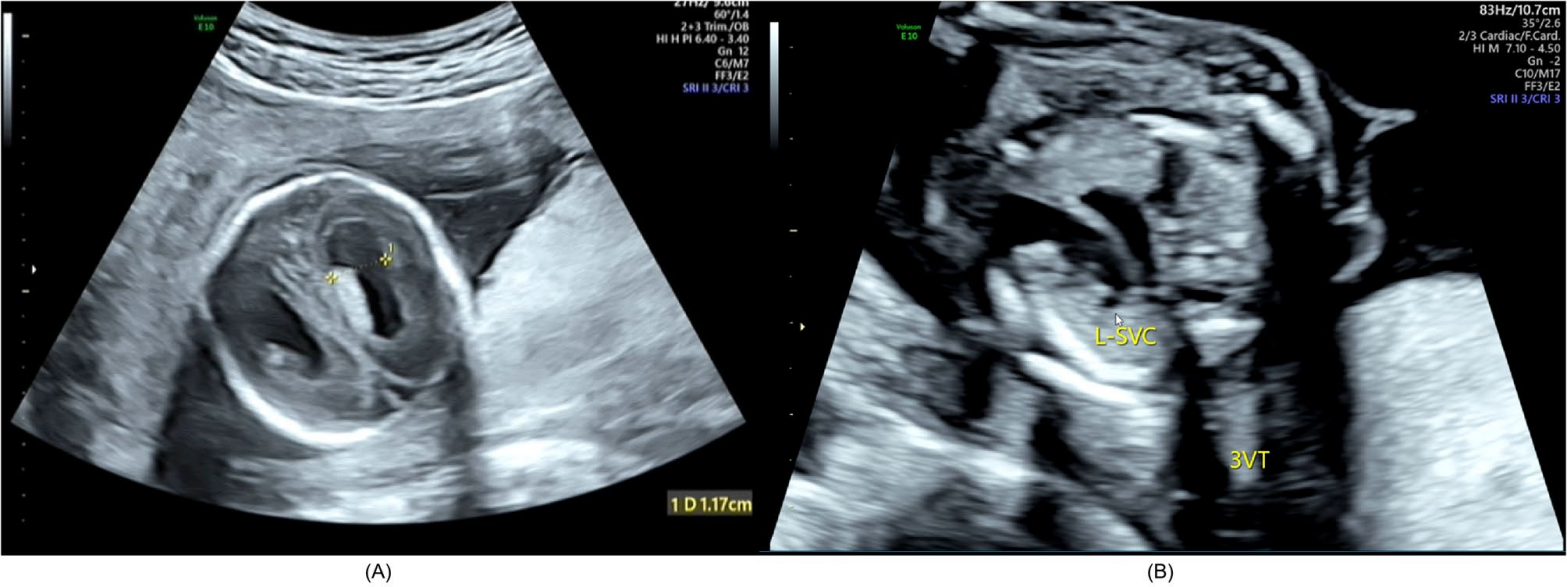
Color doppler ultrasound of II-1 during the fetal period. (A) Enlarged bilateral lateral ventricles; the dashed line 1 shows that the diameter of the lateral ventricle is 1.17 cm. (B) Persistent left superior vena cava. L-SVC: left superior vena cava, 3VT: the three vessels and trachea view
